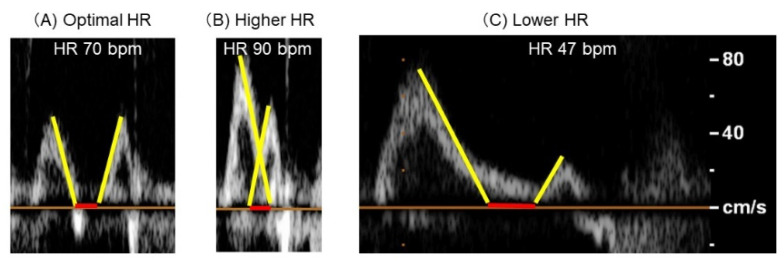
Examples of the overlap between E-wave and A-wave observed in Doppler trans-mitral echocardiographic flow. In a patient with optimal HR (HR difference ≤ ±10 bpm), E-wave and A-wave almost stand adjacent without any overlap (A). In a patient with higher HR (HR difference > 10 bpm), 2 waves stand overlapped (B). In a patient with lower HR (HR difference < −10 bpm), 2 weaves stand apart (C). HR; heart rate.
